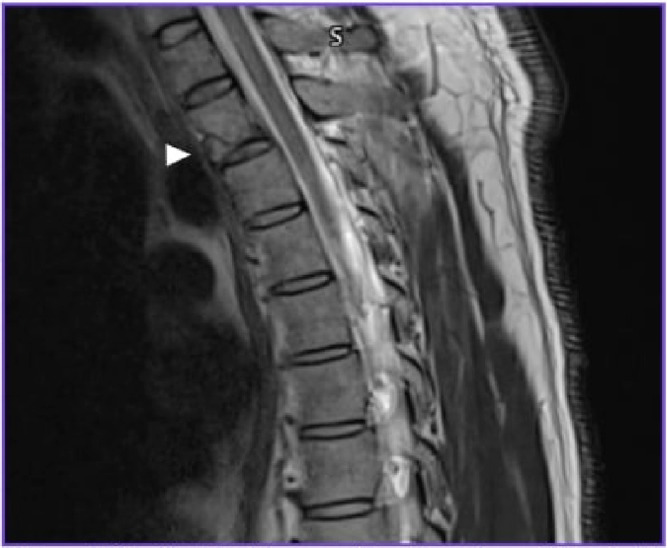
Sagittal MRI image of subluxation of the right T2/3 facet join and teardrop fracture of T2 was also noted (Arrowhead).
